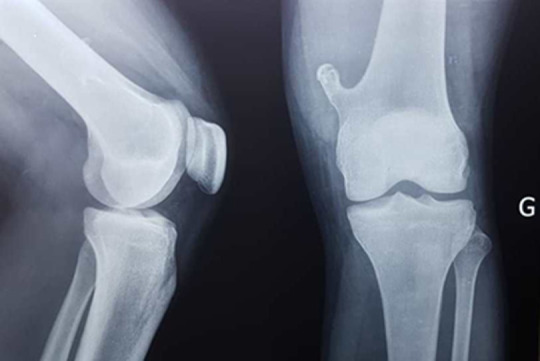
X-ray of the left knee in front and profile showing exostosis of the lower end of the femur
